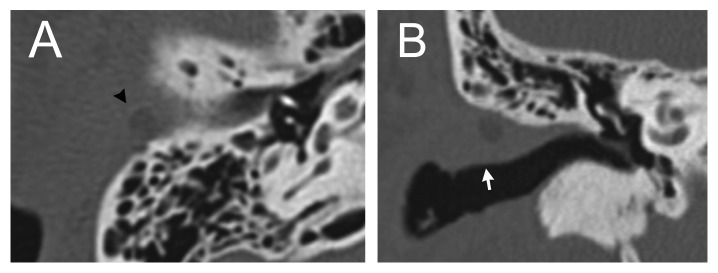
Computed tomography of the temporal bone. The axial view (A) and coronal view (B) demonstrate a dense, protruding, soft tissue lesion overlying the cartilage in the posterosuperior portion of the entry of the external auditory canal, without mastoid or middle ear lesions.
